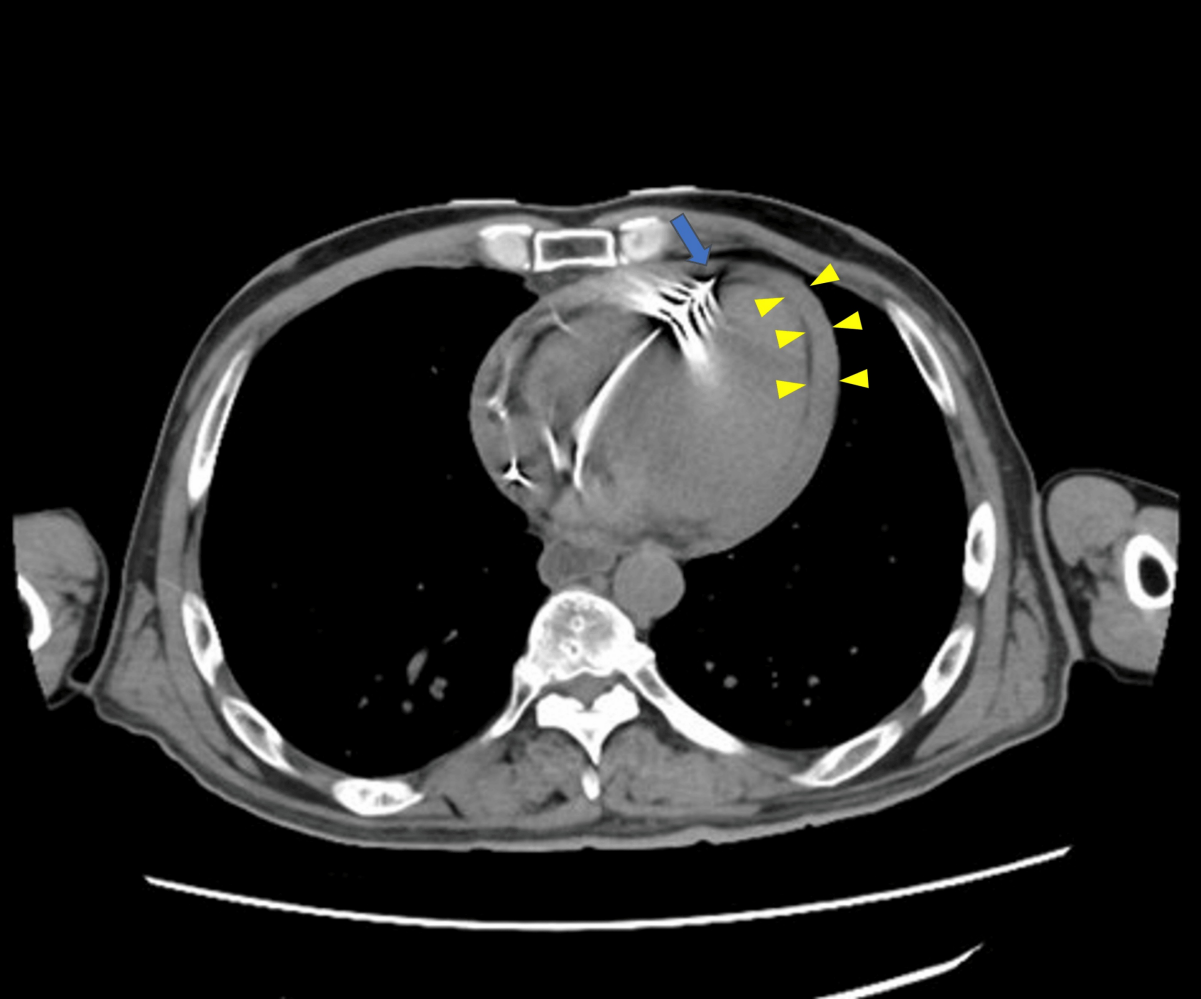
CT demonstrated deep penetration of the right ventricular lead into the right ventricular wall, although the presence of complete perforation was unclear (blue arrow); circumferential pericardial effusion around the heart was also observed in the pericardial space (yellow triangles) CT: computed tomography, RV: right ventricle
